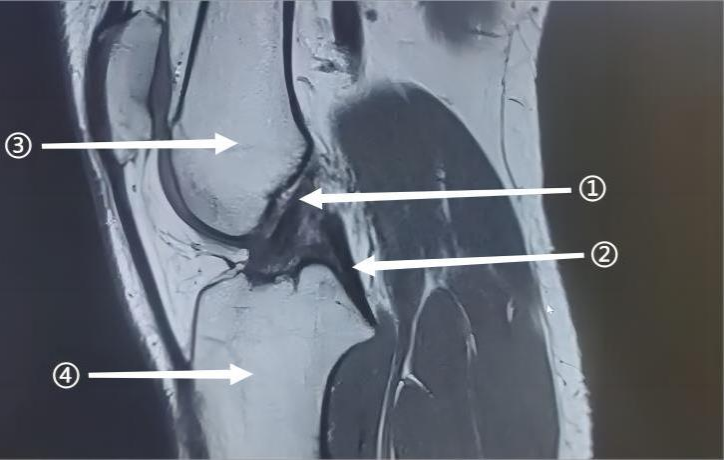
MRI sagittal view of the normal anterior cruciate ligament pathway. The figure shows: (1) Anterior cruciate ligament; (2) Posterior cruciate ligament; (3) Femur; (4) Tibia. (Source: Radiology Department, Baise People's Hospital, Guangxi, China).
